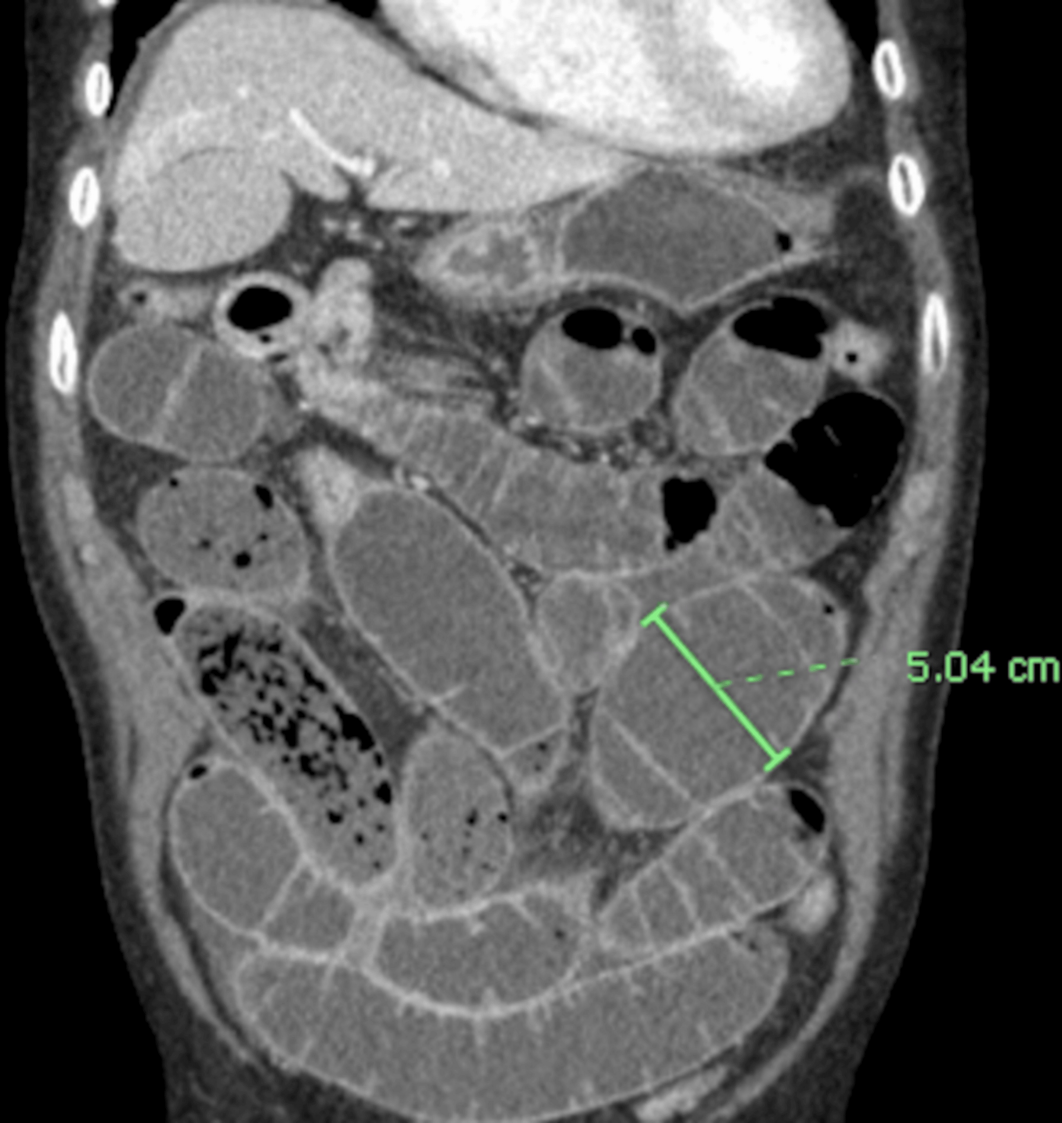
Coronal CT enterography imaging showing diffuse small bowel dilatation The small bowel demonstrates increased distension throughout, with a dilated segment measuring over 5 cm. No pneumatosis or free air is seen.
